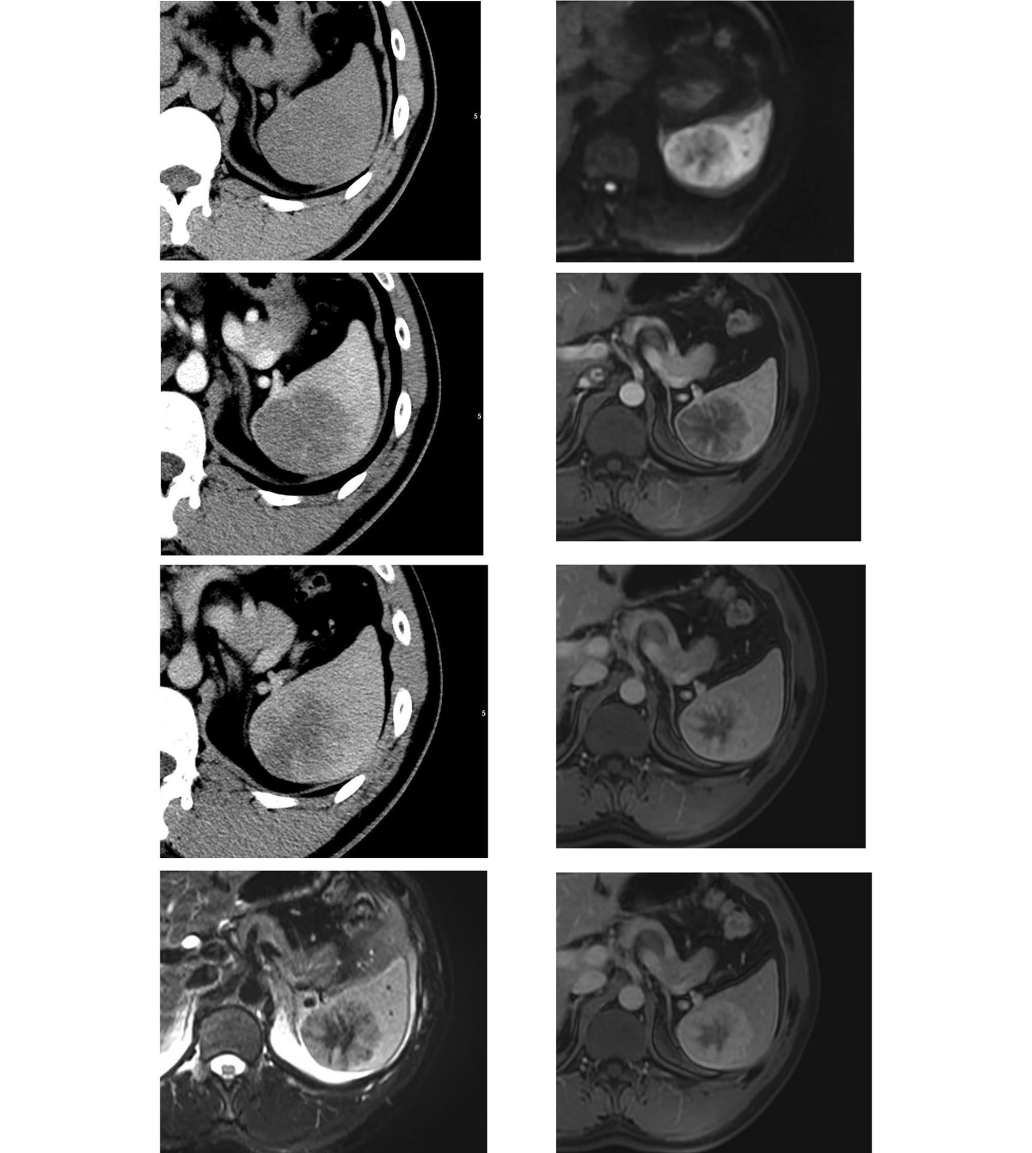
A middle-aged male with no specific clinical symptoms was diagnosed with SANT. Unenhanced axial CT image (2 A) shows a hypodense lesion in spleen. On contrast-enhanced CT images(arterial phase:2B and portal phase:2 C), the lesion shows progressive and centripetal enhancement. The lesion shows hypointensity on T2WI(2D) and DWI(2E), with central more hypointense scars. After contrast administration, enhancement with the pattern of spoke-wheel is seen during arterial phase(2 F), portal venous phase(2G) and delayed phase(2 H)
